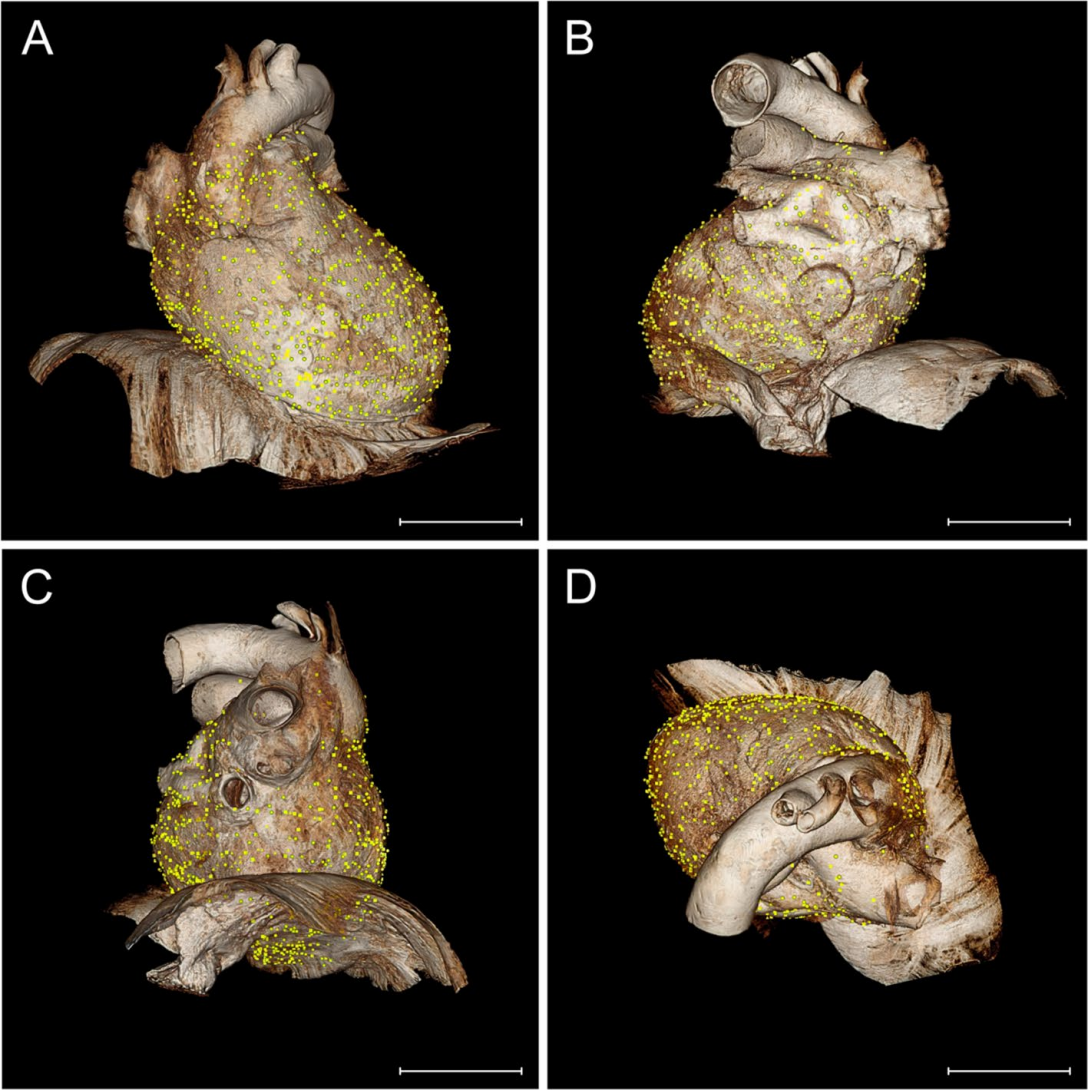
Distribution of Ruffini-like corpuscles (RLCs) in the human pericardium. Mapping and visualization of the proprioceptors were performed by digitally transferring all RLCs of the eight pericardia studied to a three-dimensional volume-rendering model used as a "standard- pericardium”. This three-dimensional model was acquired by magnetic resonance imaging from a formalin-fixated heart, including the pericardium and diaphragm of a 91-year-old female body donor. Transfer of all receptors was implemented using 3D-Slicer software. Each receptor location was mapped according to anatomical landmarks and relative to size, considering the adjacent environment and cardiac topography. Symbols represent the three pericardial localizations of corpuscles (triangle = 1st localization, circle = 2nd localization, quadrate = 3rd localization). A total number of 1476 RLCs was detected. Scale bars: 5 cm. A Distribution of RLCs as seen from the front of the pericardium. B Distribution of RLCs as seen from the posterior side of the pericardium. C Distribution of RLCs as seen from the left and lower sides of the pericardium. D Distribution of RLCs seen from above the pericardium
